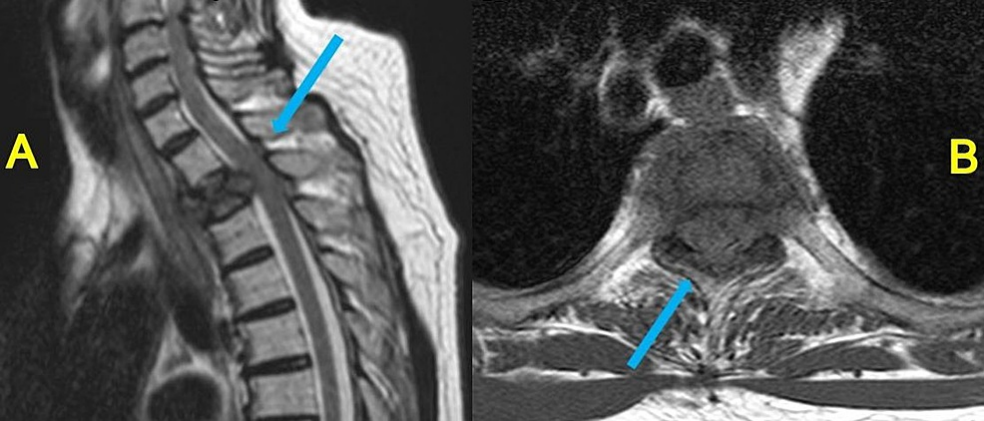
MRI at disease onset shows metastatic spinal cord compression in the second thoracic vertebra. A:　T2-weighted image (sagittal)
